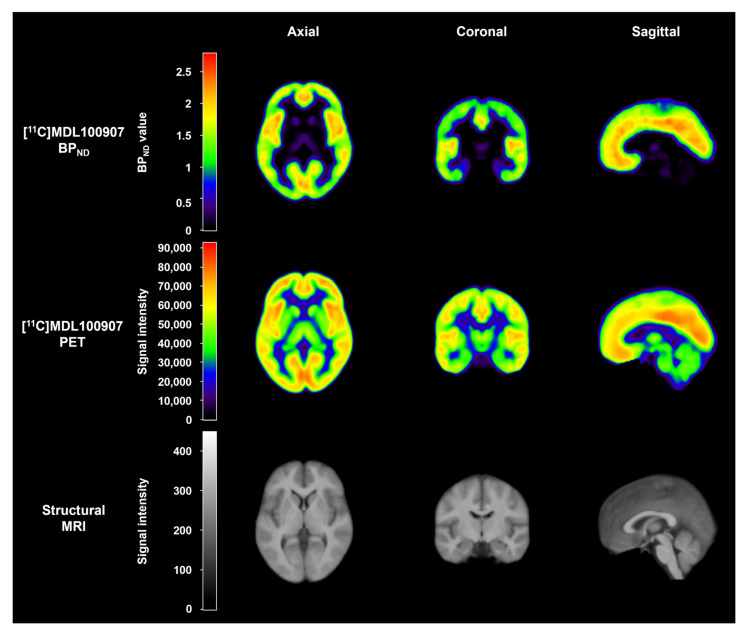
Representative mean images of [^11^C]MDL100907 BP_ND_, [^11^C]MDL100907 PET, and corresponding structural MRI in 33 healthy subjects. BP_ND_, binding potential with respect to non-displaceable compartment; PET, positron emission tomography; MRI, magnetic resonance imaging.
